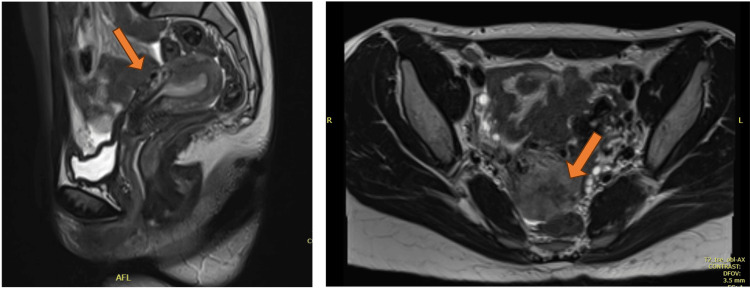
Pelvis MRI with contrast post-uterine artery embolization showing a thrombsed arteriovenous malformation in the right parametrium and left parametrial serpentine vascular channels with contrast enhancement, suggestive of persistent activity.
